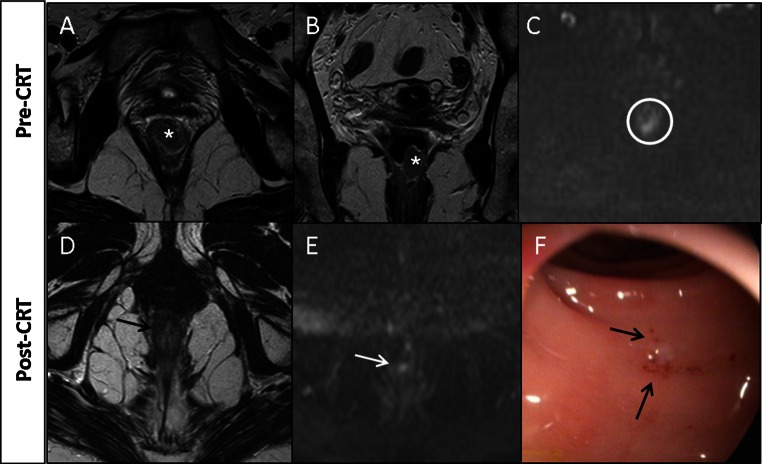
a, b Distal tumor (*asterisks*) before CRT at T2W-MRI and c DWI. After CRT at T2W-MRI (d) and DWI (e), residual tumor was suspected (*arrows*). At endoscopy (f), CR (*arrows*) was determined, and the patient was treated with wait-and-see policy. After 3 months, DWI became normal; patient remained free of recurrent disease at 3.8 years of follow-up
